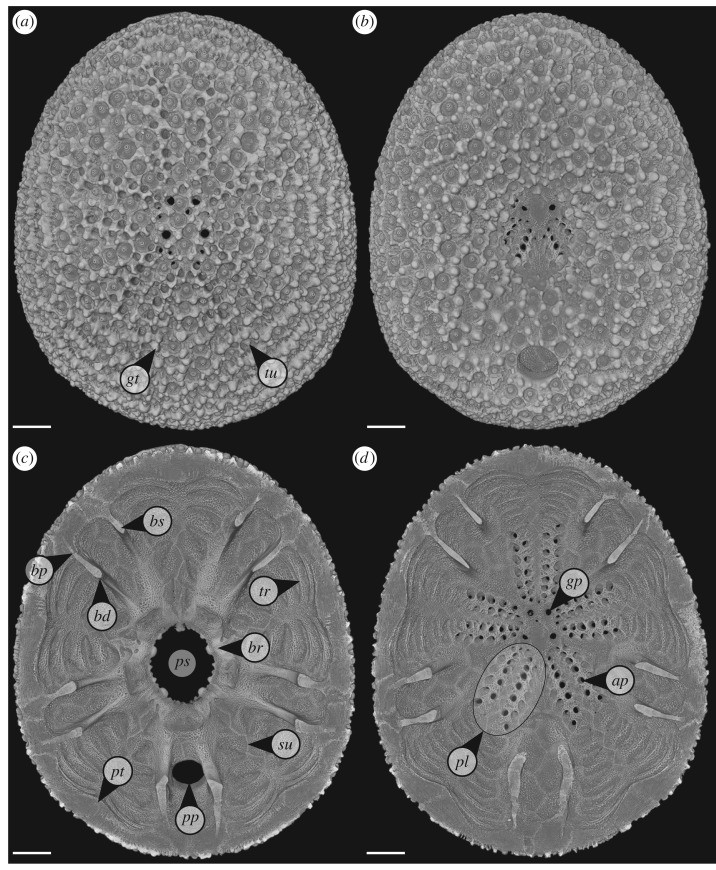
Micro CT rendering of *E. pusillus* from Giglio island, Italy. (*a*) Skeleton in aboral view. The ambulacral system and microstructures such as the tubercles and glassy tubercles are visible. (*b*) Skeleton in oral view with the prominent peristome and periproct visible. (*c*) Top view of the oral side. The internal supports are visible, as well as the basicoronal ring around the peristome. (*d*) View on the inner part of the aboral side. Pores and the limits of the buttresses are visible. *ap*, ambulacral pore; *bd*, distal region of the buttress; *bp*, proximal area of the buttress; *br,* basicoronal ring; *bs*, buttress; *gp,* genital pore; *gt,* glassy tubercle; *pl,* petal; *ps*, peristome; *pt*, plate thickening; *pp,* periproct; *su,* suture; *tr,* transversal ridge; *tu,* tubercle. Scale bar, 500 µm.
